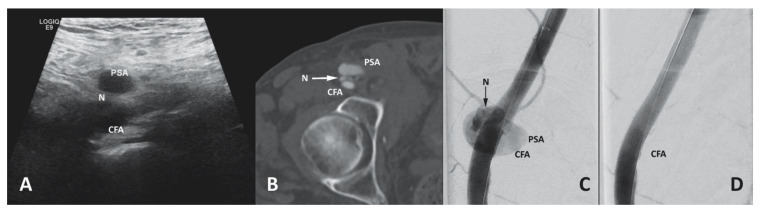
(A) Sonographic, (B) computer-tomographic, and (C) fluoroscopic evidence of pseudoaneurysm formation (PSA) with a short, broad neck (N) in the common femoral artery (CFA) after transfemoral THV implantation. (D) Pseudoaneurysm exclusion by endovascular implantation of a balloon-expandable covered stent graft (8 × 50 mm Gore^®^ Viabahn^®^).
